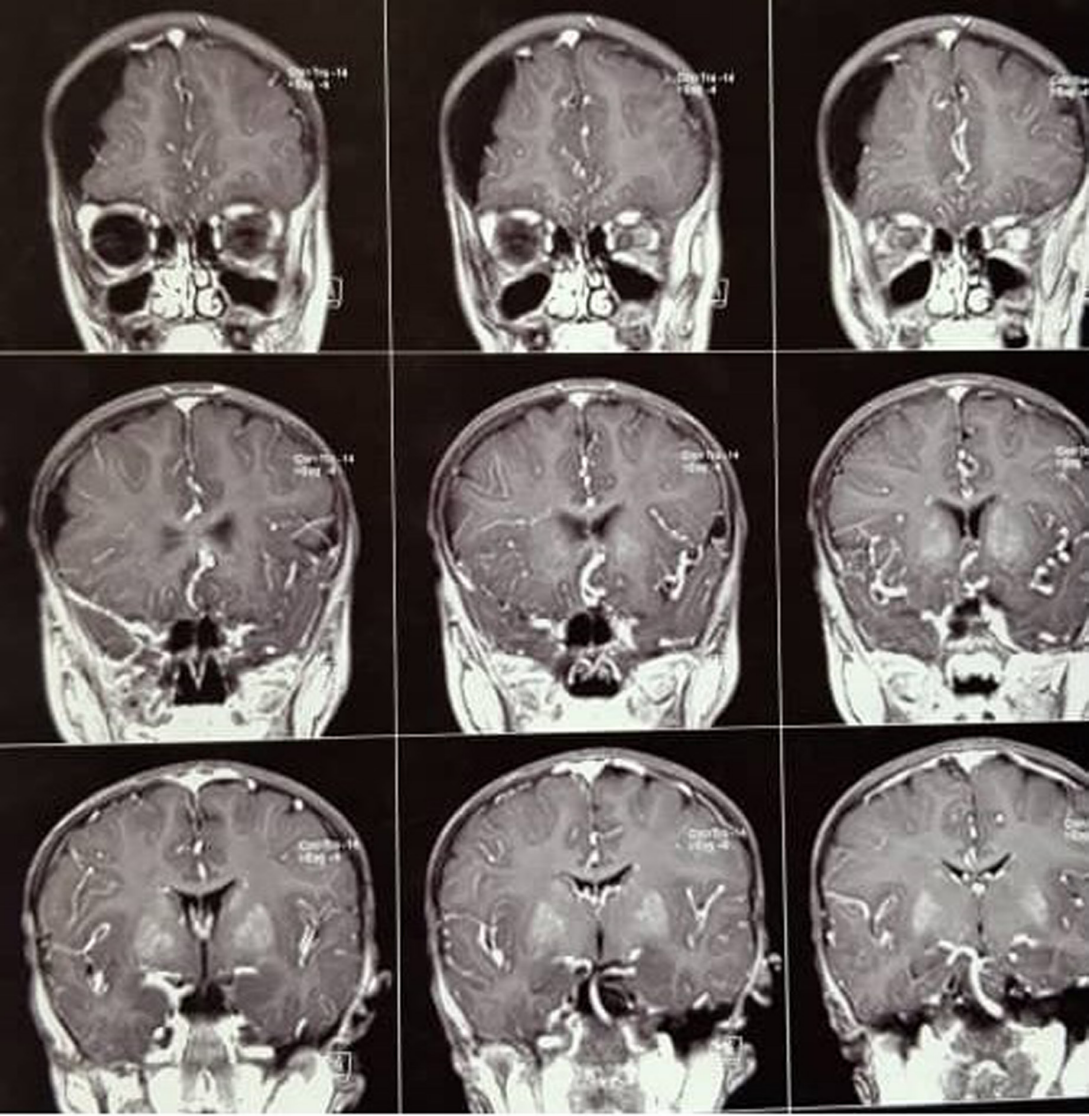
Coronal planes of brain MRI of the proband case demonstrate symmetrical high signal intensities in putamen and a subdural effusion in right side
